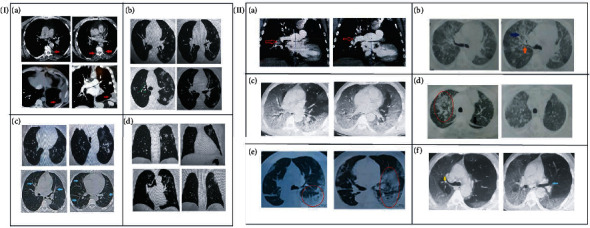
(I) Computerized tomography (CT) axial image of COVID-19 patients had moderate illness showing (a) plural effusion at the lower lobe (red arrow); (b) faint ground-glass opacity (GGO) and multiple nodular (green arrow); (c) tree-in-bud pattern (turquoise arrow), bronchiectasis, and air trapping; and (d) coronal image showing plural effusion. (II) Computerized tomography (CT) angiography coronal image of COVID-19 patients who suffered from severe illness showing (a) pulmonary embolism in the right pulmonary artery (red arrow); (b) axial image showing fibrotic bronchiectasis (blue arrow) and nodular air bronchogram (orange arrow); (c) diffuse massive bilateral GGO with tree-in-bud pattern; (d) massive consolidation (red circle) with bands in the lower right and left lobes (black arrow); (e) massive consolidation (red circle) in the left lobe; and (f) diffuse GGO in the right lobe and pulmonary nodules (yellow arrow) and air bronchogram (blue arrow).
